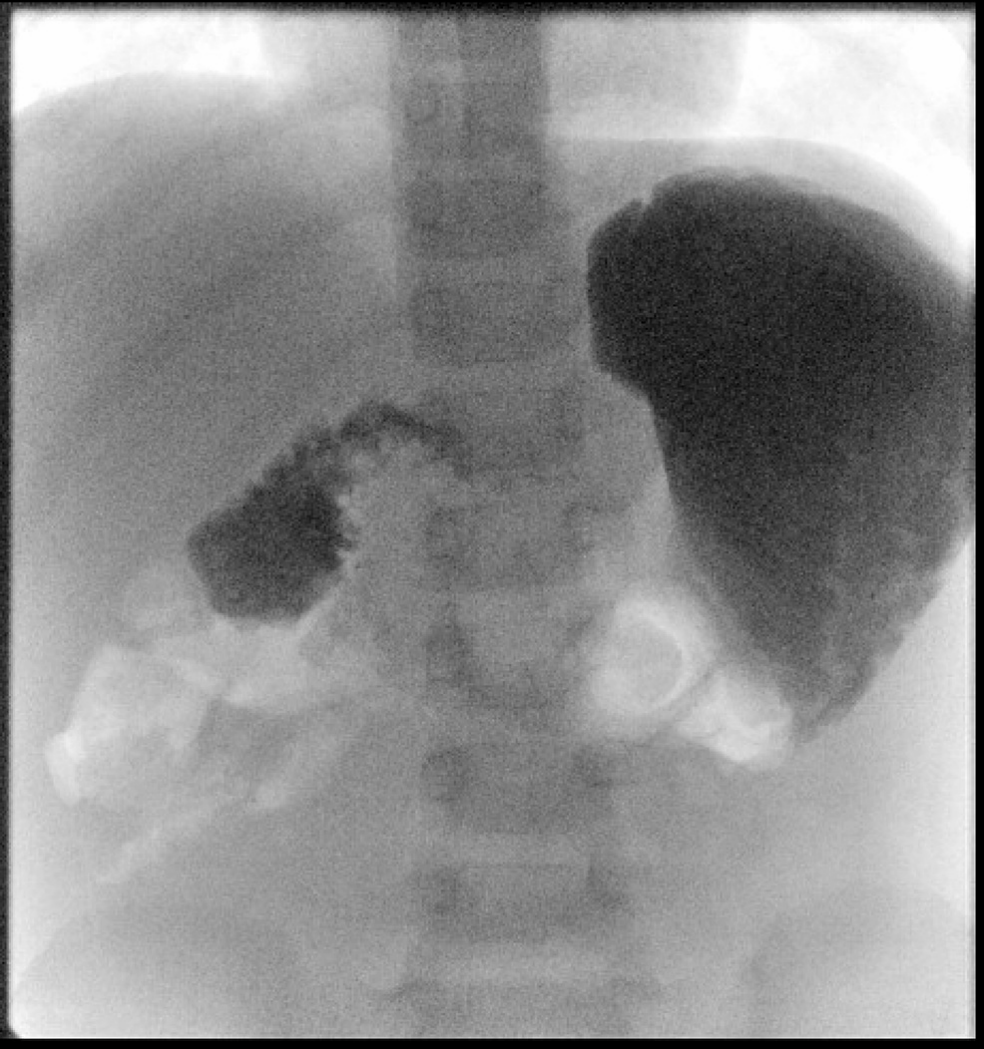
Water-soluble barium contrast X-ray revealing obstruction in the duodenum at D2
